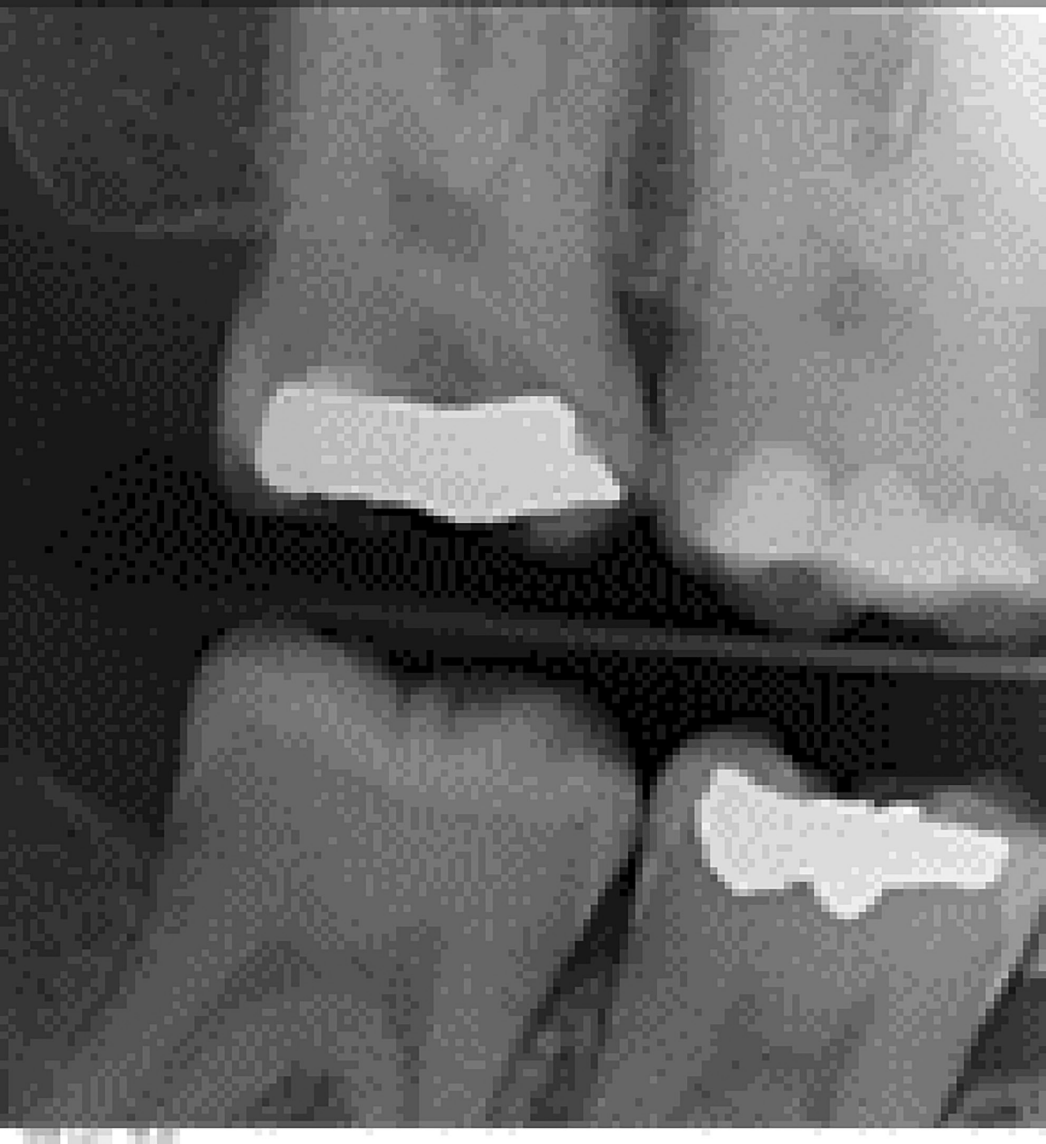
Preoperative periapical radiograph.
